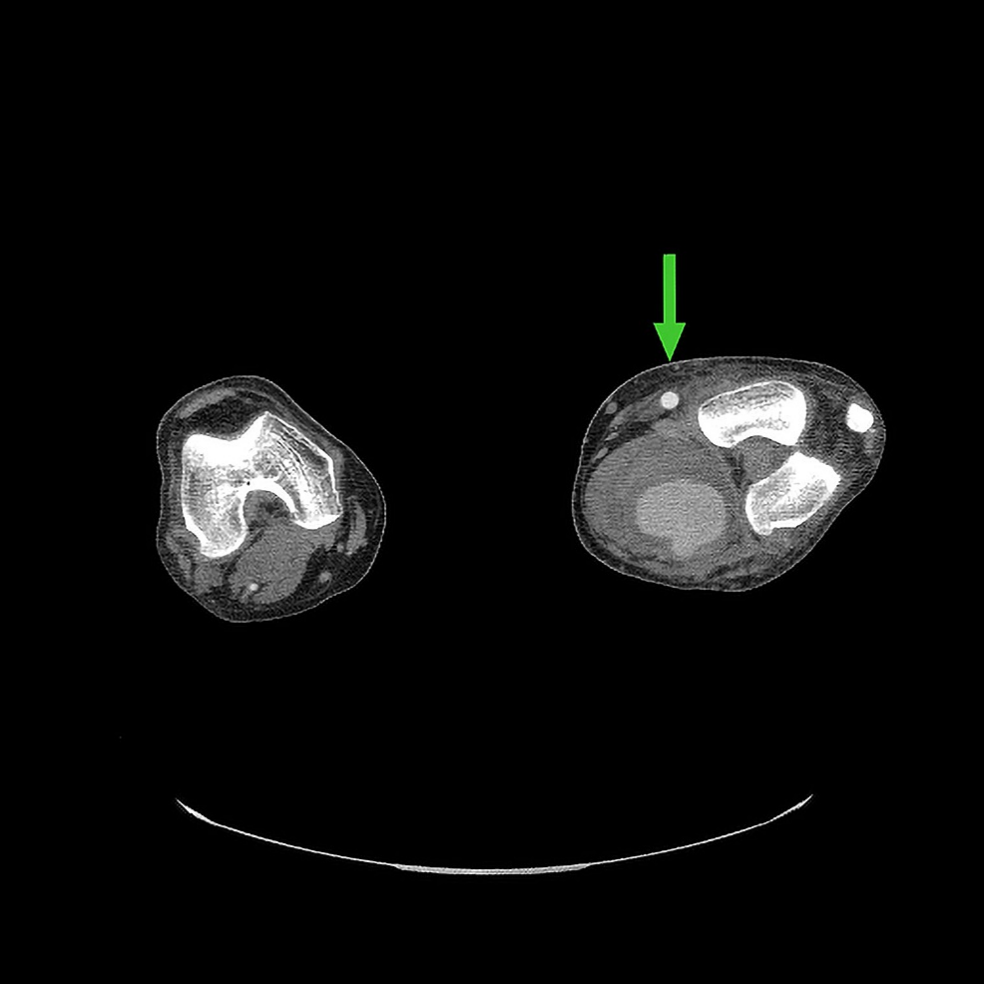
Postoperative CT scan image of left lower extremity The image shows an interval increase in the size of ligated popliteal aneurysm to 9 cm filling the left popliteal fossa. A patent bypass graft can be easily seen at 12 o’clock (arrow) immediately above the aneurysm CT: computed tomography
